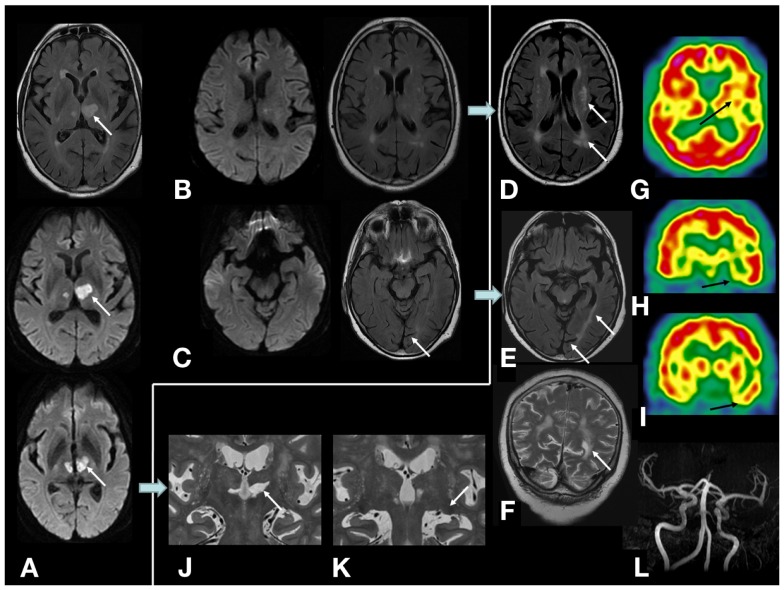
On MRI examination, initial diffusion-weighted, and FLAIR sequences showing (A) initial bi-thalamic infarction predominantly on the left side; (B,C) no lesion of optic radiations, temporal, and parietal lobes was observed in the acute phase of stroke; (D–F) 1 year and 8 months later, left white matter hyperintense lesion appeared in the left external capsule and semiovale center and temporo-parieto-occipital white matter, cortical and subcortical atrophy on the left occipital lobe (calcarine sulcus). No occipital or parietal lesion occurred; (G–I) cerebral perfusion SPECT (^99^Tc-HMPAO) showed hypoperfusion in the left striatum, thalamus, perisylvian, and temporal cortex; (J,K) lesion of left medial and lateral thalamic nuclei. Lateral geniculate body and pulvinar were not damaged; (L) no intracranial stenosis were found on time-of-flight MR angiography.
